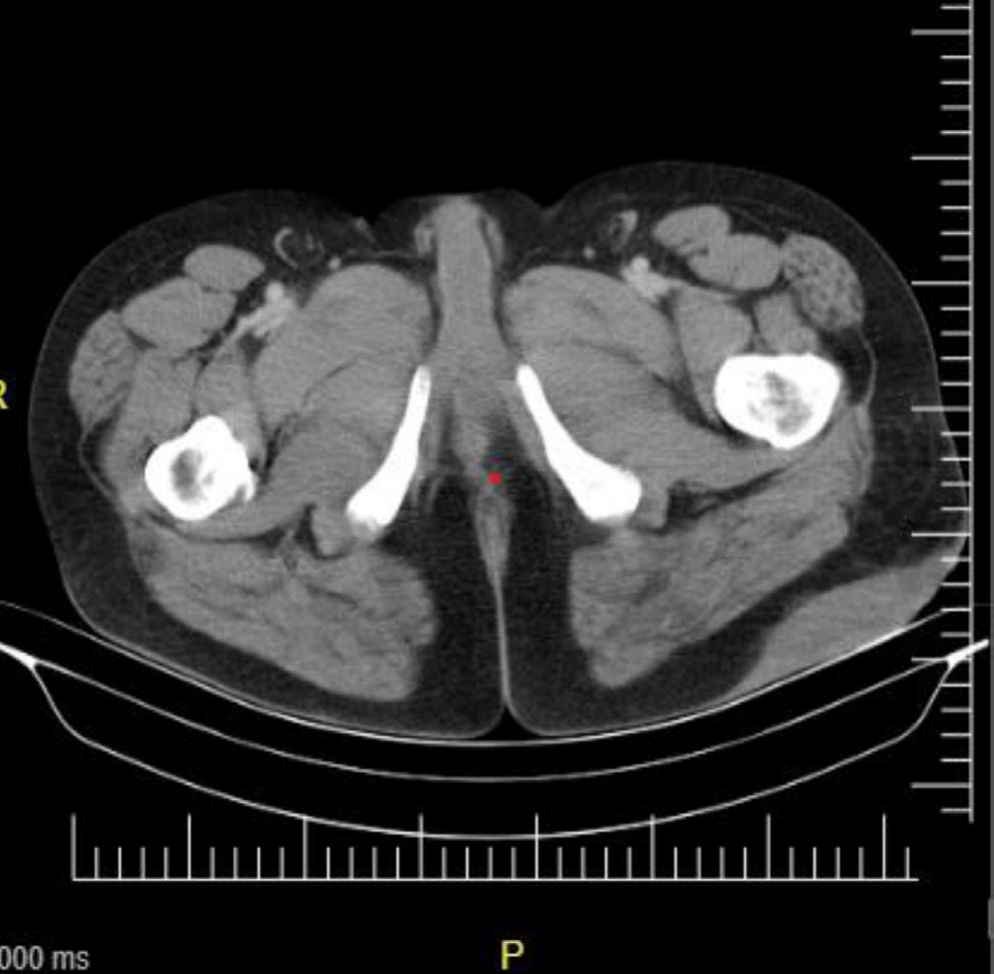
Computed Tomography (CT) scan of the patient revealing subcutaneous thickening in the left gluteal and proximal thigh regions, consistent with a soft tissue infection.
